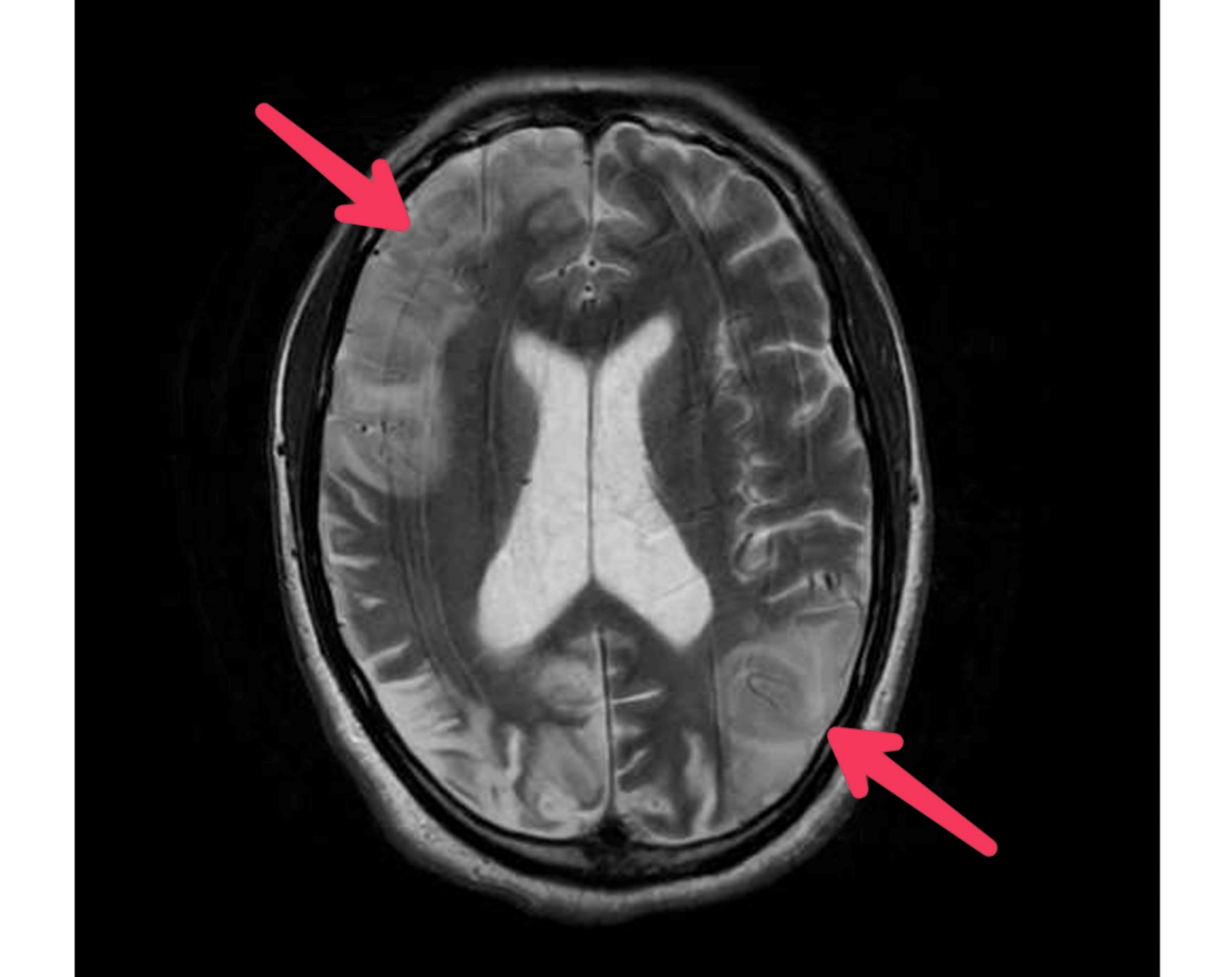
Acute infarcts as seen on initial MRI of the head
